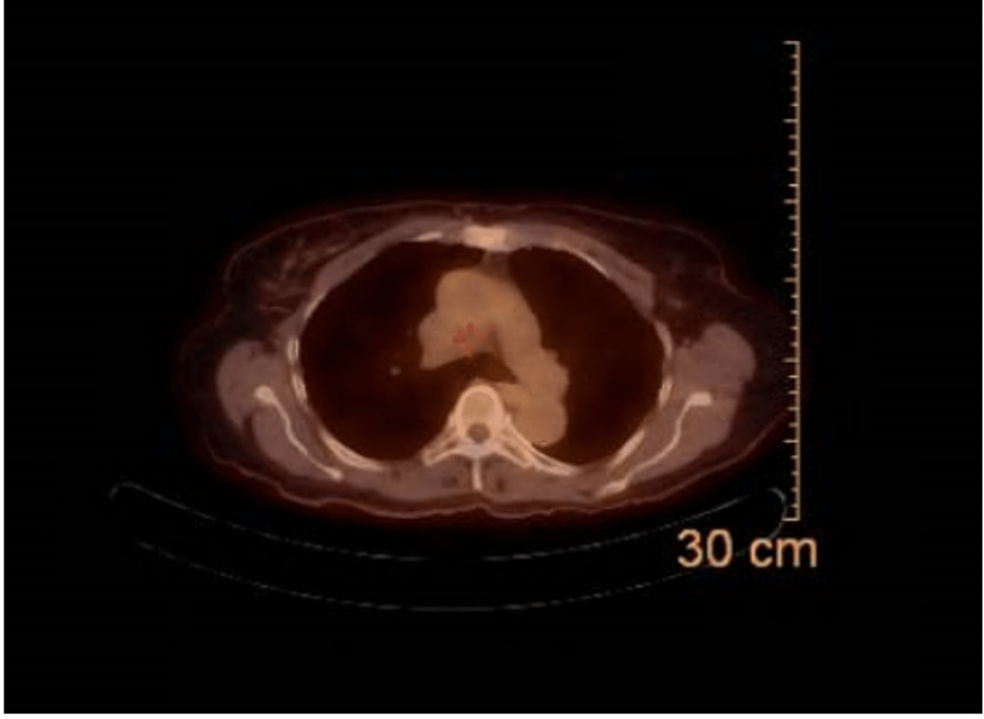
PET-scan, did not show any FDG PET: positron emission tomography; FDG: fluorodeoxyglucose
